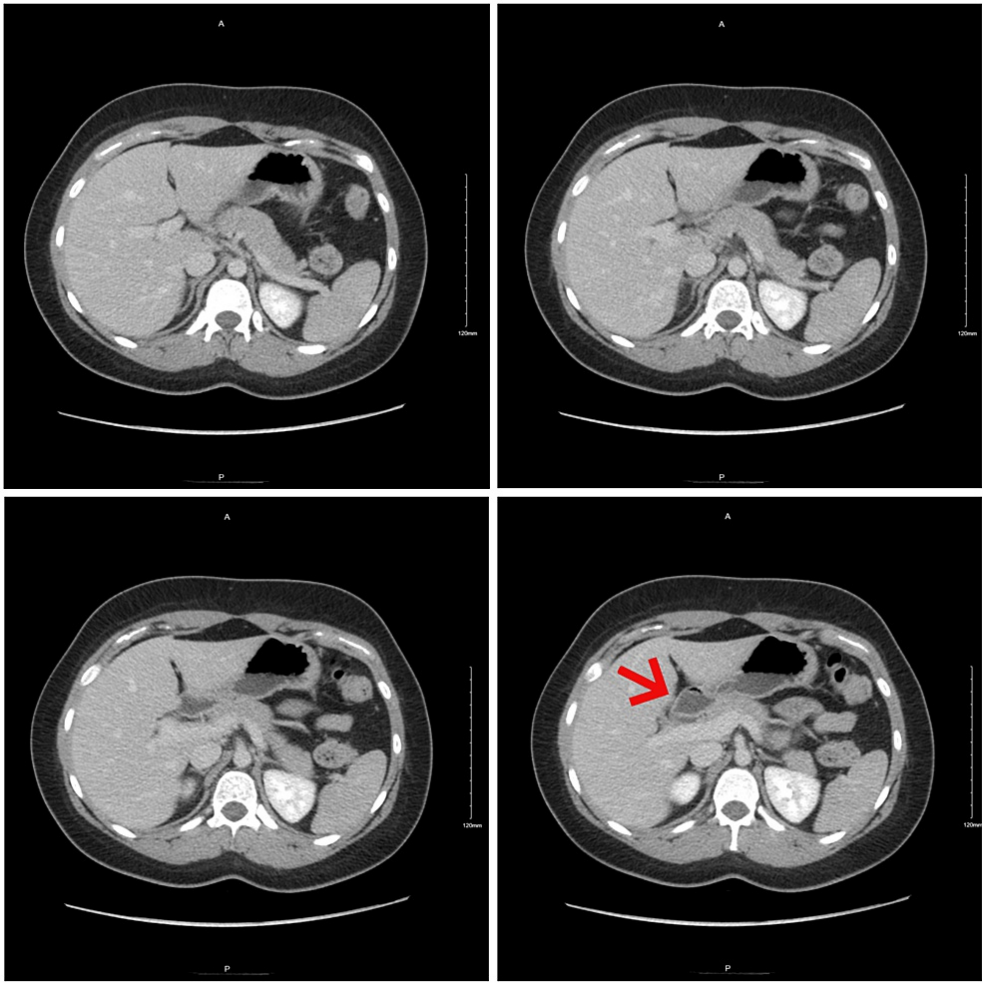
Abdominal and pelvic CT with contrast. We can see the structure thought possibly representing a left-sided gallbladder, which is more consistent with the post-pyloric duodenum (arrow). CT: computed tomography.
